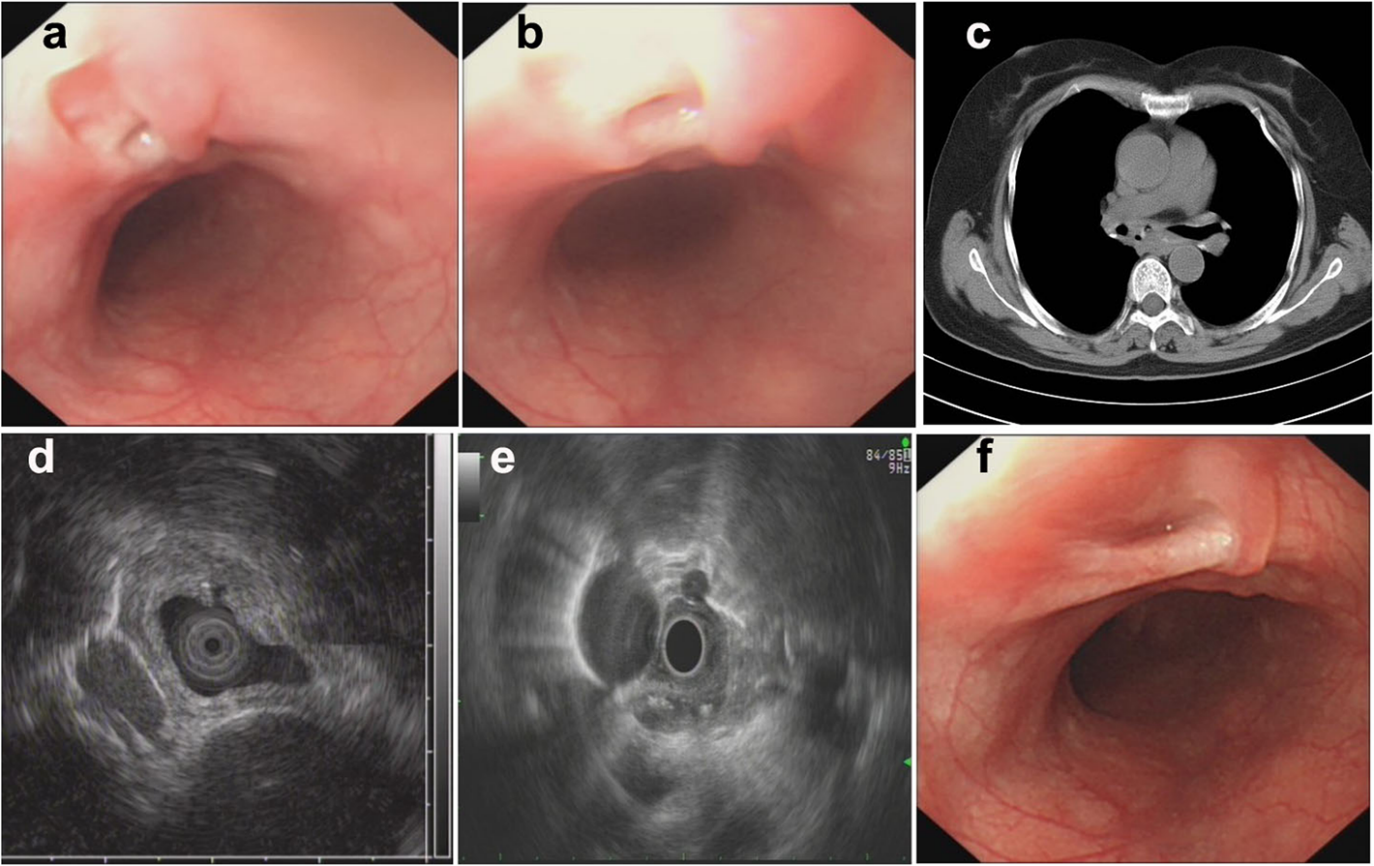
Esophagoscopic findings and EUS features of pathologically undiagnosed ET (case 8). a (far view) and b (near view) Esophagoscopy: an ulcer with suspicious fistula formation. c, chest CT: a small amount of bubbles and fluid in the mediastinum around the esophagus. d and e, EUS: thickening, poorly defined esophageal wall structure, with one or more enlarged paraesophageal lymph nodes. f, repeat esophagoscopy after treatment: a white mucosal depression with scar formation
